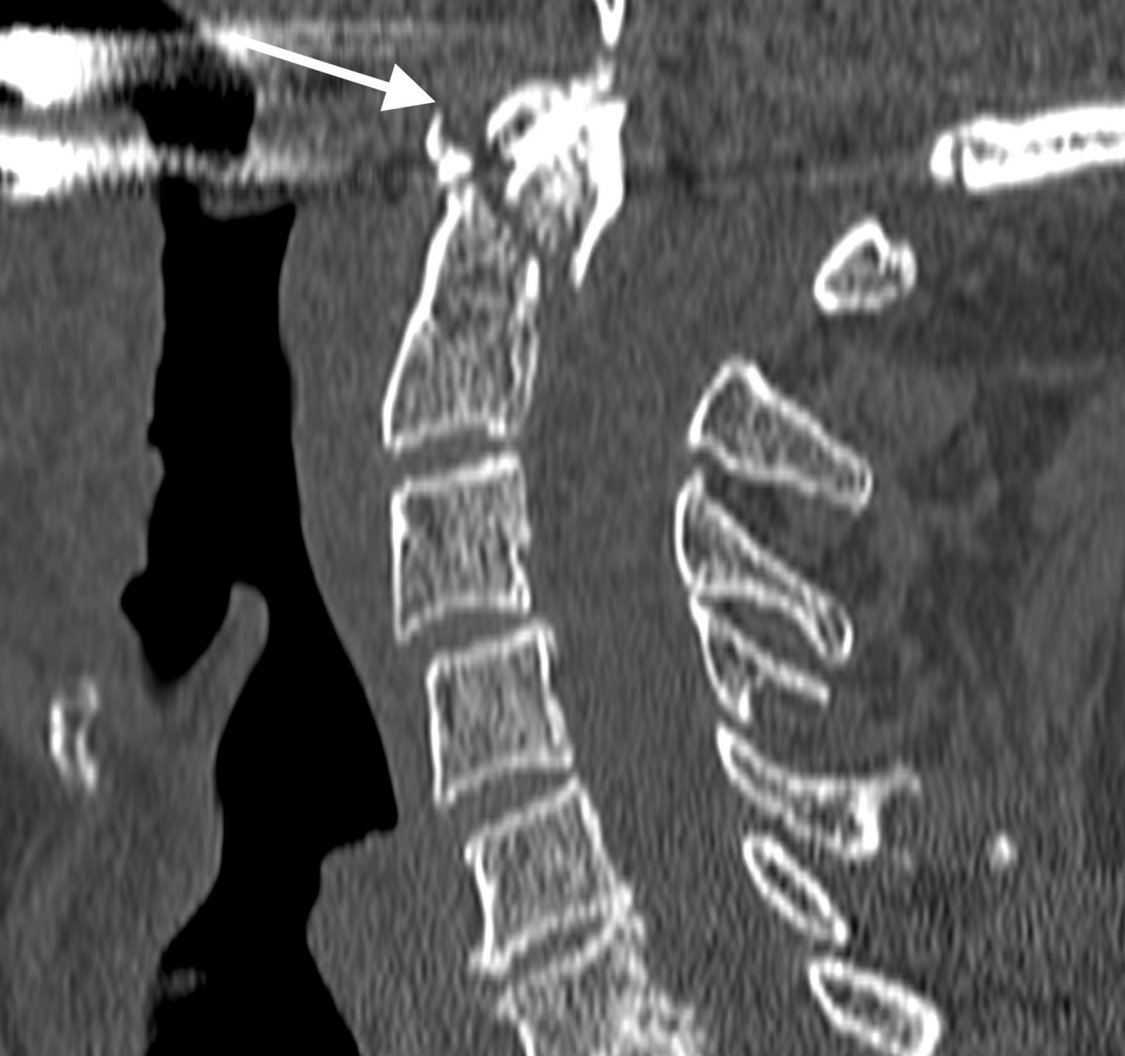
Computed tomography (CT) scan, odontoid fracture Sagittal CT scan showing a type II odontoid fracture. A 3 mm displacement is observed (arrow).
